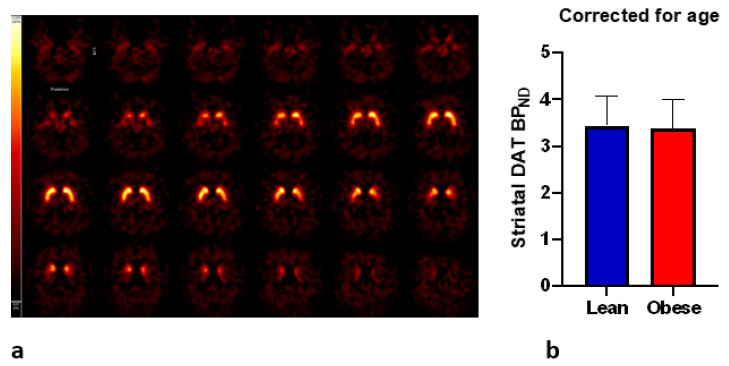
Striatal DAT BP_ND._ (a) A representative example of SPECT image of the brain (transversal slices) acquired 3 h after administration of ^123^I-FP-CIT in a lean subject. (b) Striatal DAT BP_ND_ (mean ± SD) did not differ significantly between lean subjects and subjects with obesity after controlling for age (one-way ANCOVA).
